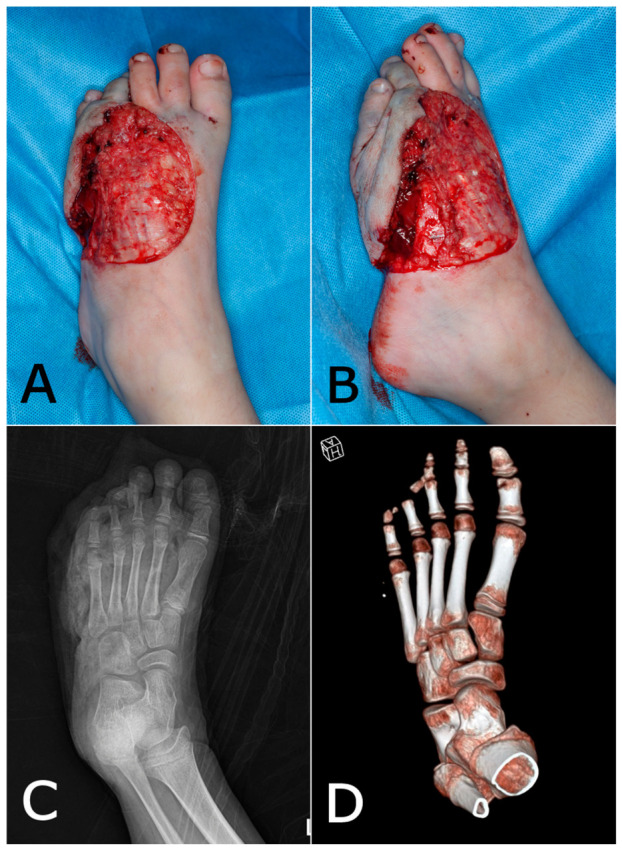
Preoperative clinical photographs, radiograph, and three-dimensional computed tomography image. (A,B) Preoperative clinical photographs demonstrating a degloving injury of the dorsal aspect of the left foot. (C,D) Preoperative radiograph and three-dimensional computed tomography image showing a fracture of the proximal phalanx of the great toe, with dislocation of the fourth proximal interphalangeal and fifth distal interphalangeal joints.
